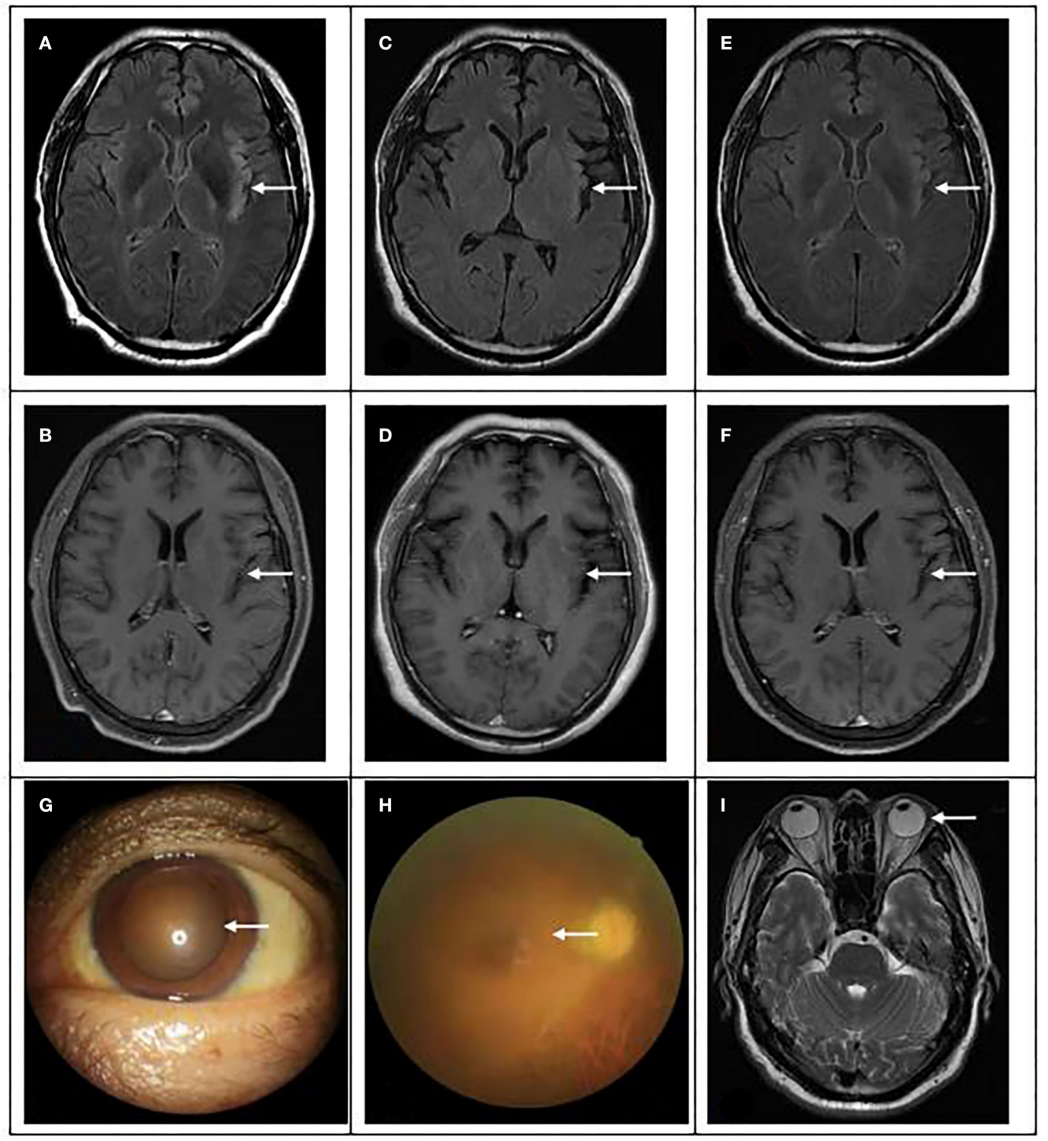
Brain magnetic resonance and eye examination results. (A,B) On 15 April, brain magnetic resonance showed multiple spots and bands of abnormal signals in the left insular and temporal cortex, high signal in T2WI and T2flair, and equal and low signal in T1flair. (C,D) On 24 April, MRI showed abnormal signal and meningeal changes in the left frontal temporal insula. (E,F) On 04 June, magnetic resonance imaging showed that the range of inflammatory lesions was slightly smaller than before. (G) The pupil of the patient was significantly enlarged, with a diameter of 5 × 5 mm. (H) Fundus lesions: vitreous cavity is turbid, the optic disc boundary is indistinctly seen, and white line changes can be seen in the peripheral momentum. (I): No abnormality was found in eyeball magnetic resonance.
